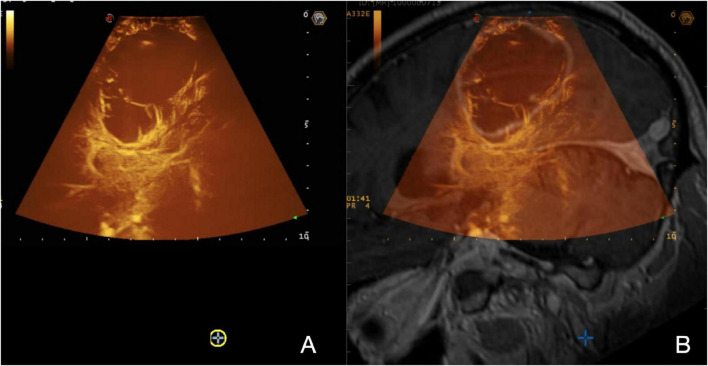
Navigated MIP-CEUS. (A) On the left, a real-time MIP-CEUS sequence is displayed; (B) on the right, the corresponding pre-operative MRI is fused with real-time ioUS. The signal obtained throughout the MBs distribution can be depicted in a MIP image to describe the entire vascular-tree including smaller vessels under structural and functional aspects, giving detailed representation of the CE pattern.
